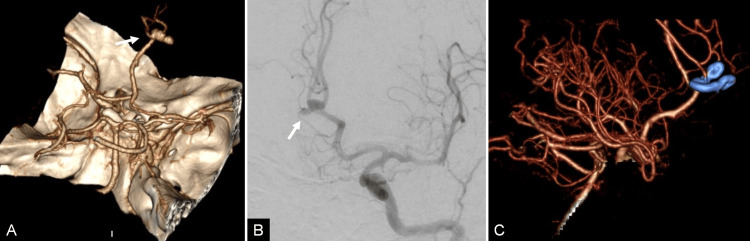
Pre- and postoperative radiological studies of case 1 (A) Preoperative computed tomography angiography (CTA) with 3D reconstruction and (B) left carotid angiogram demonstrating a trilobulated aneurysm (arrow) arising in the azygos bifurcation. (C) Postoperative CTA with 3D reconstruction showing complete obliteration of the aneurysm.
